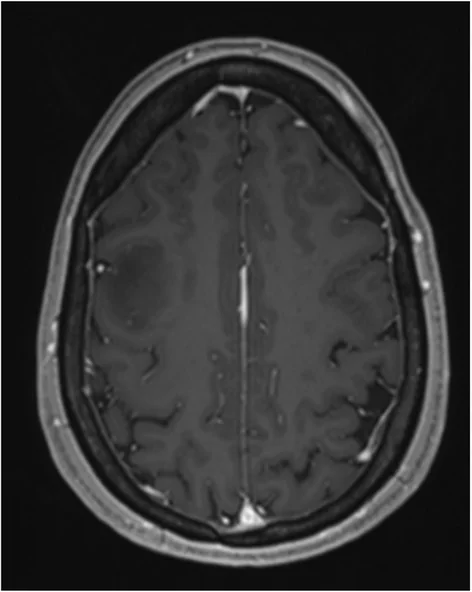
Axial T1‐weighted brain MRI shows a hypointense mass predominantly located in the white matter but focally extending into the precentral gyrus of the right frontal lobe.
